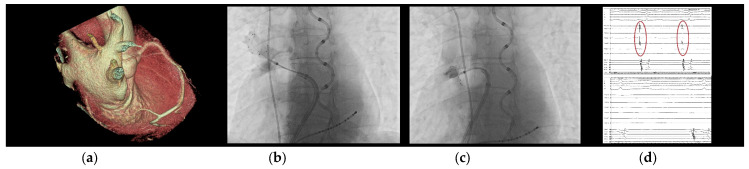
CB ablation of an additional branch of the RSPV, with a complex take-off angle. From left to right: (a) CT imaging of this additional RSPV branch, vertically oriented. (b) Unsuccessful attempt to occlude and isolate this branch with the 28 mm size CB. (c) Successful attempt to occlude and isolate this branch with the 31 mm size CB. (d) POLAR map recorded electrograms in this additional RPV branch, pre- and post-cryoenergy delivery.
